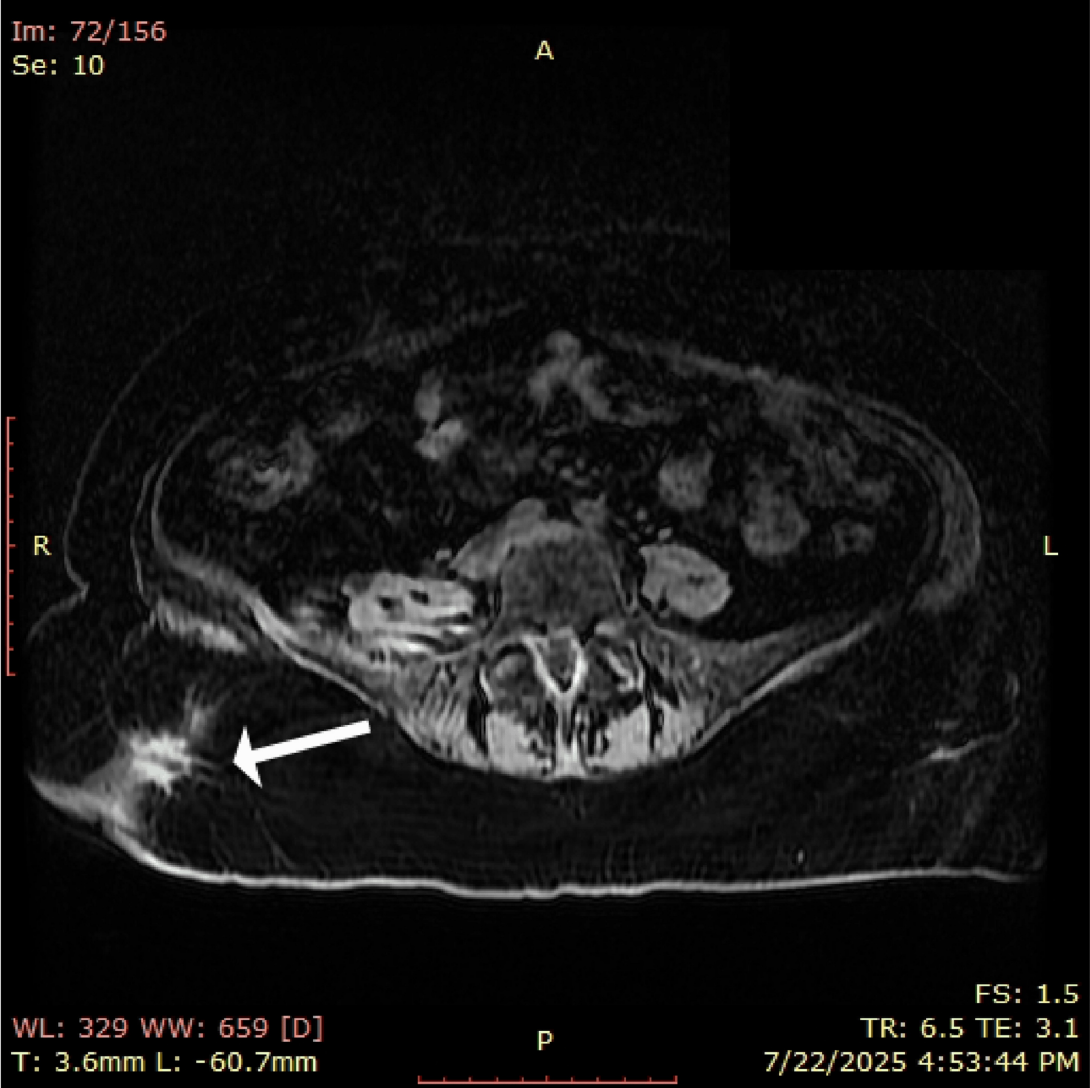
Preoperative lumbar spine MRI showing multiloculated paravertebral collection (white arrow).
